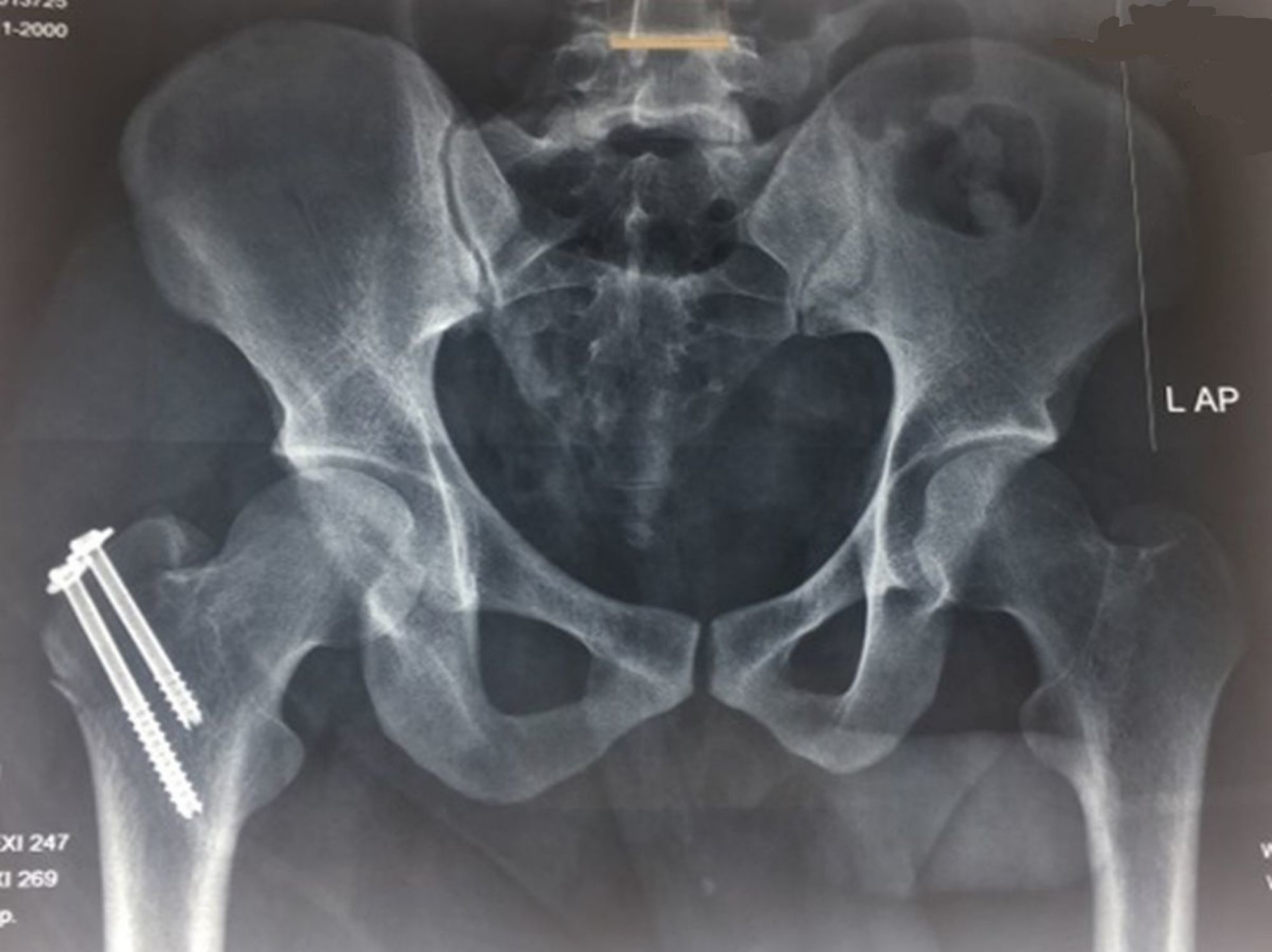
Post-operative plain radiograph of the pelvis with bilateral hips – anteroposterior view Fixation of greater trochanter fracture with two 6.5 mm cannulated cancellous screws with washer, along with congruent reduction of the right hip joint.
